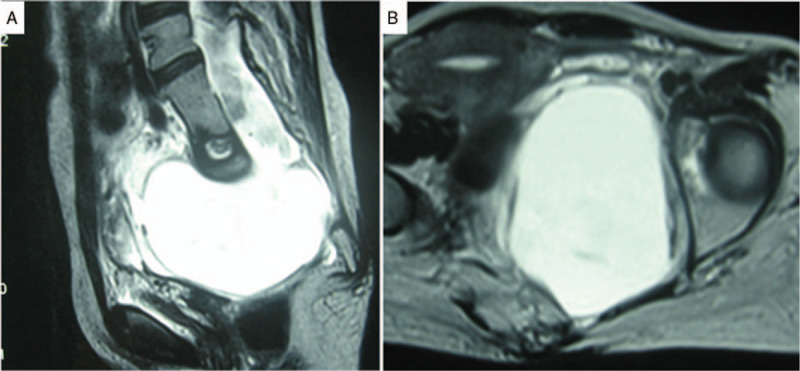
Anterior sacral meningocele in patient 2. Sagittal (A) and axial T2-weighted MRI (B) shows a large, well-defined cystic mass.
